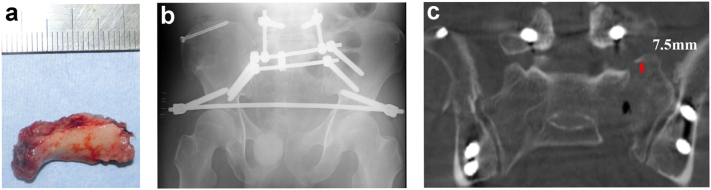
a: Extracted spinal canal fragment that measured 25 mm × 10 mm. b: Postoperative plain radiograph shows the iliac tips and internal fixator screws intersecting at the acetabulum. c: Postoperative computed tomography scan shows vertical reduction from 15 mm to 7.5 mm.
